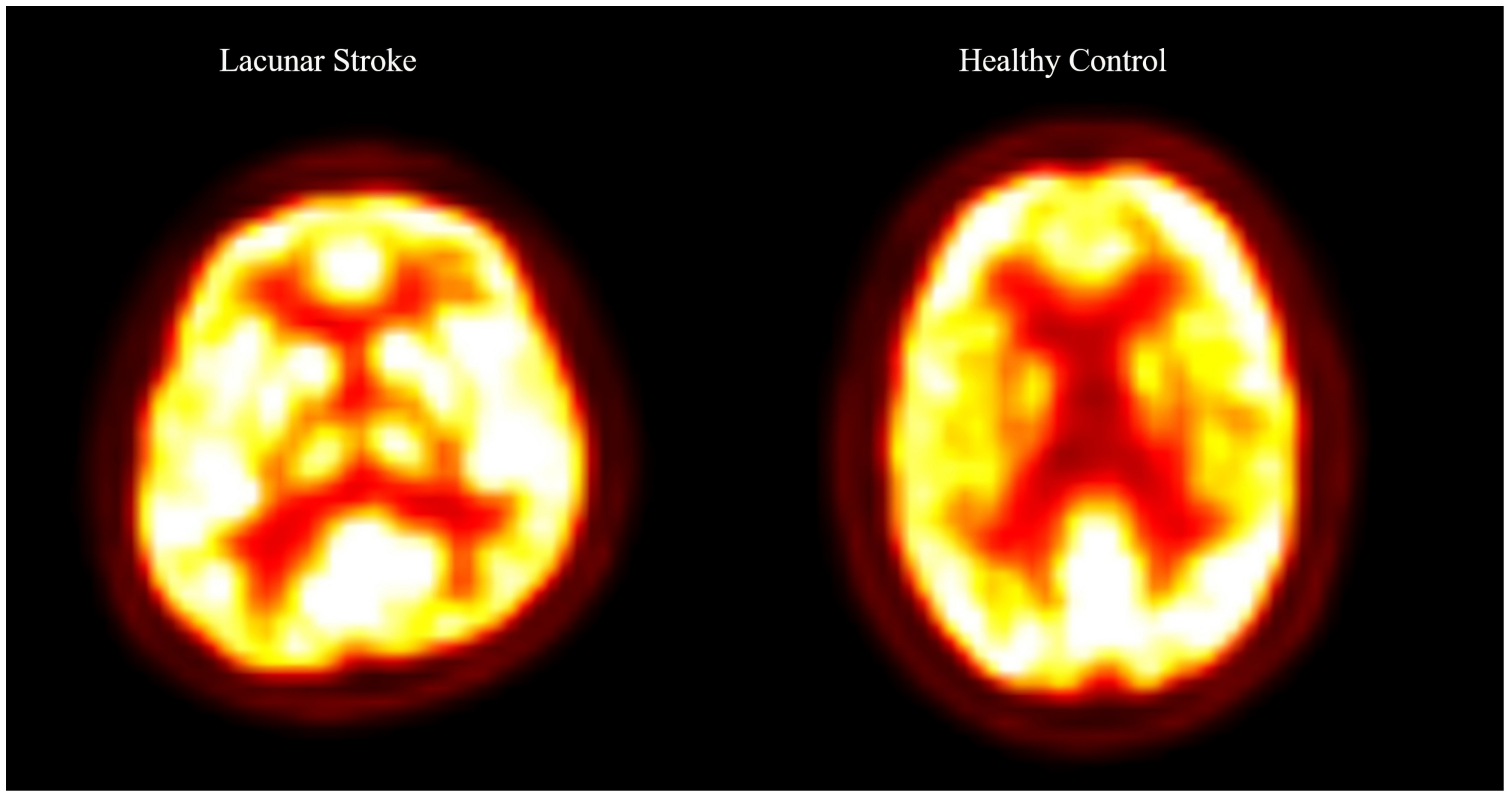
Representative ^18^F-FDG PET/MRI images from a patient with lacunar stroke (LS) and a healthy control (HC).
